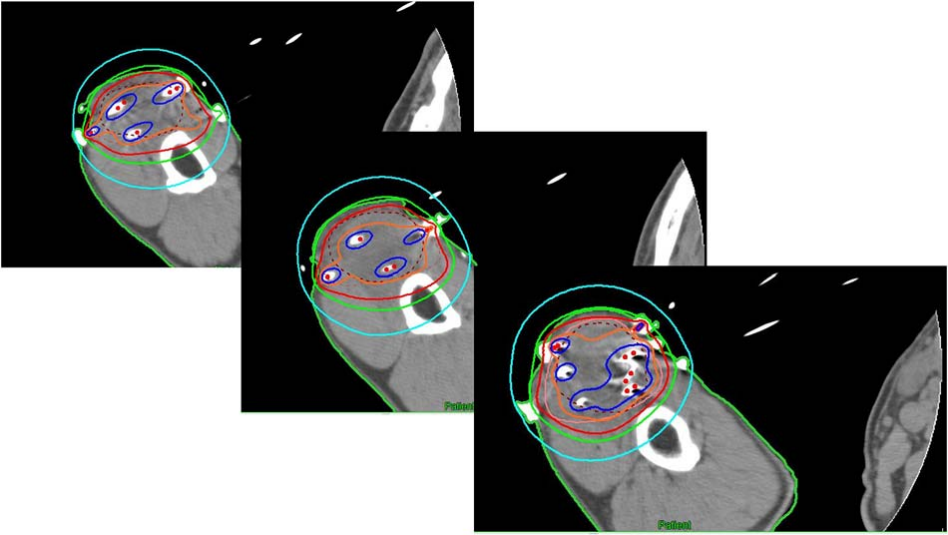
The dose distribution of HDR-ISBT of the right upper arm on the axial image of the planning CT. The red, orange and blue lines represent 100, 150 and 200% isodose lines, respectively. The red dotted line represents the gross tumor volume at the time of brachytherapy.
